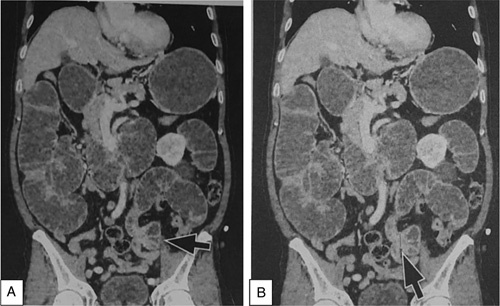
Abdominal computed tomography scan showing an internal hernia. (A) Intramesosigmoid hernia at the distal jejunum (arrow). (B) Neck of intramesosigmoid hernia (arrow).
